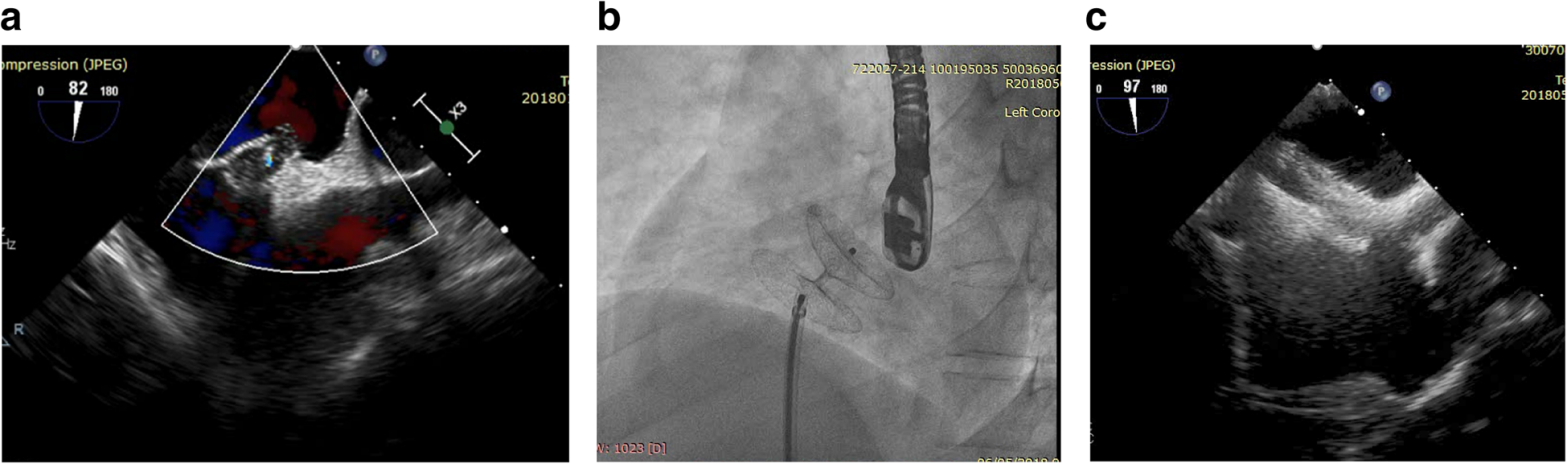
a 2D trans-esophageal echocardiography in bicaval view showing balloon sizing of the patent foramen ovale (PFO). b LAO view showing the cribriform device while deployment. c 2D trans-esophageal echocardiography in bicaval view showing cribriform device in place
